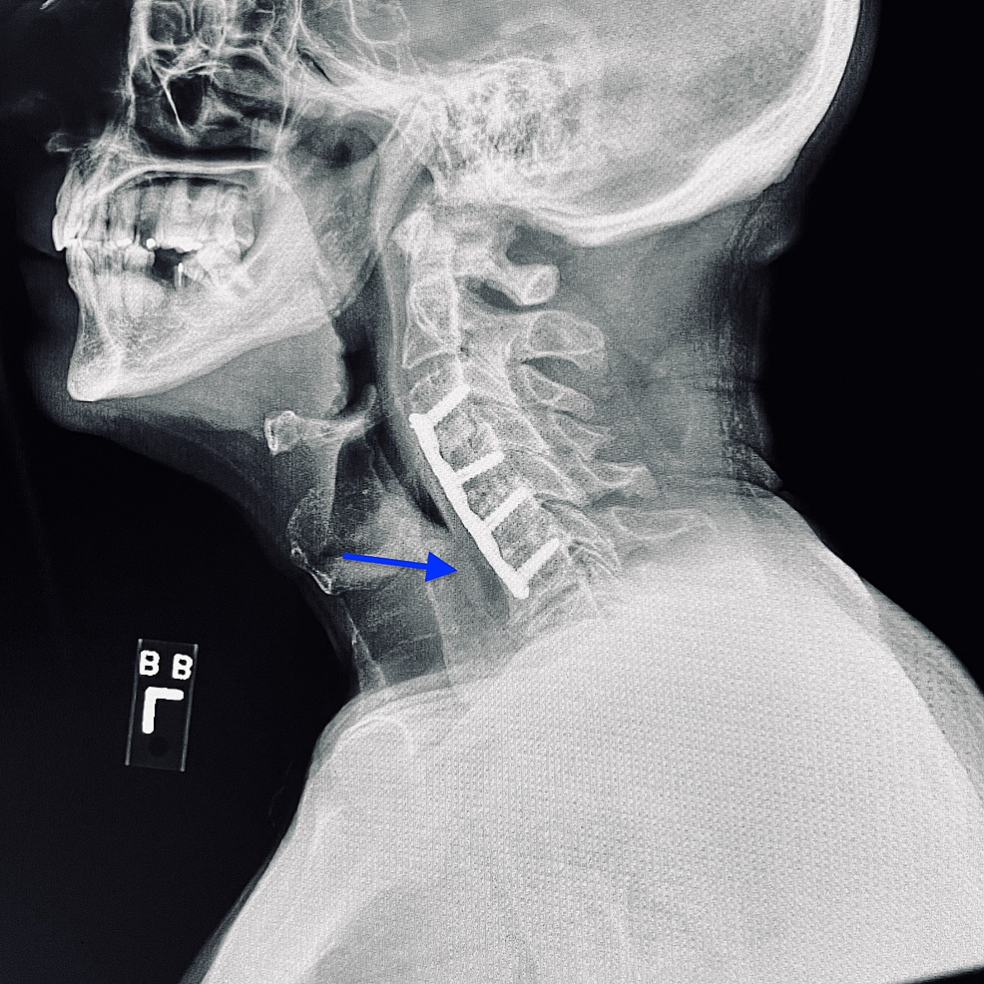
Lateral view of X-ray neck showing mild distension of the proximal esophagus (arrow)
